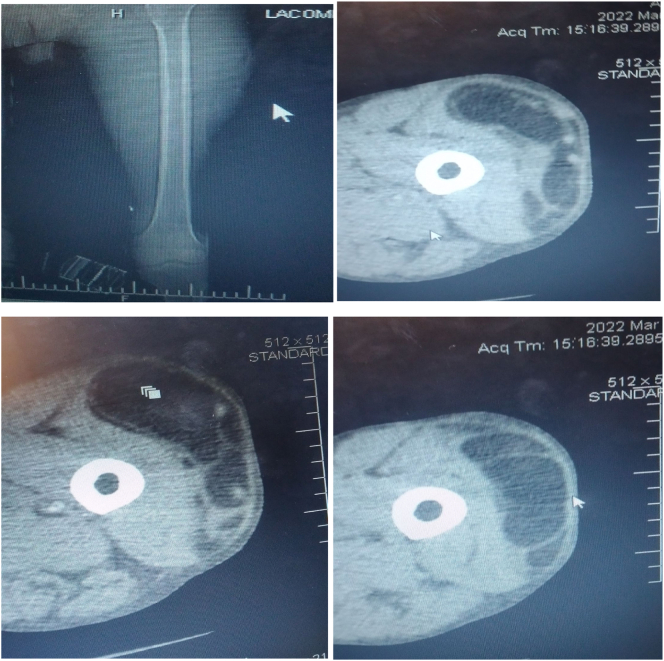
A-D: Cross-sectional CT-images of the mass demonstrating hypodense lesion with heterogenous, irregular and septated lesion.
